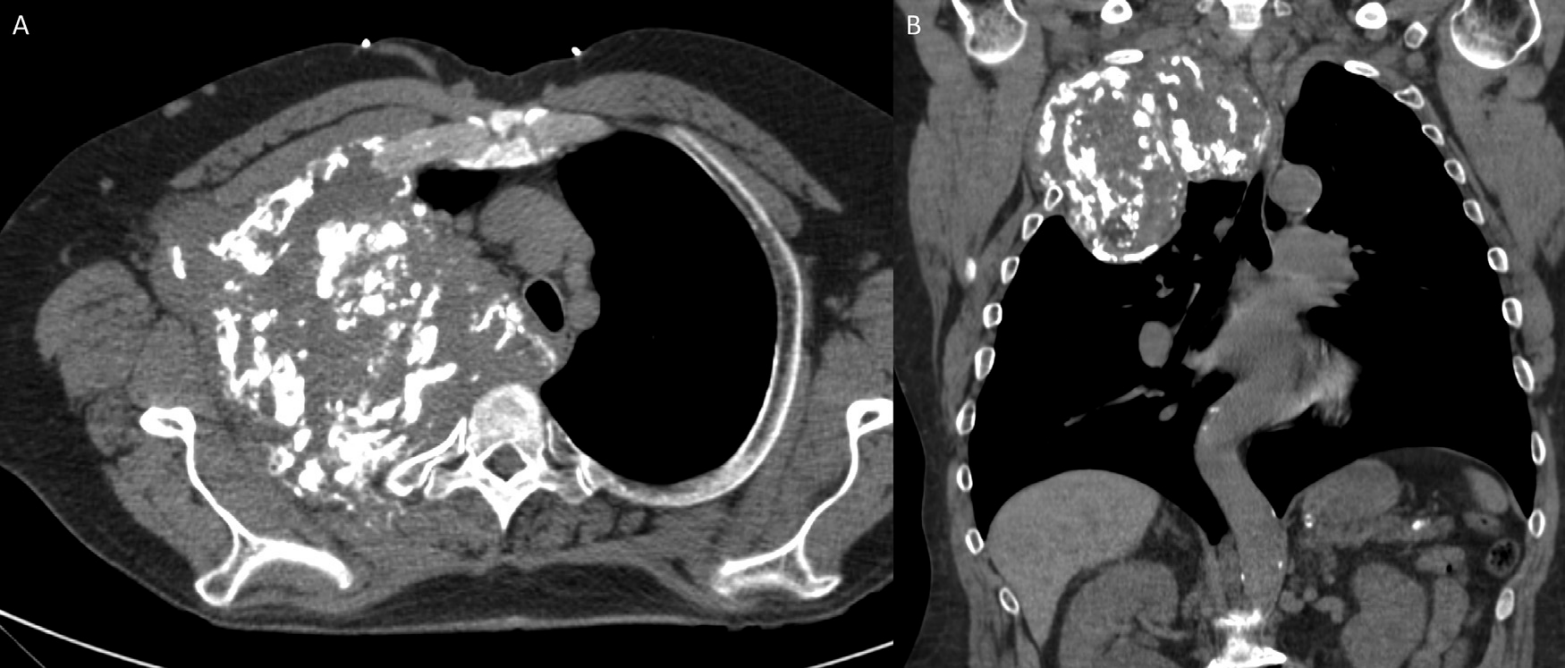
Unenhanced CT of the chest, axial (A) and coronal (B) demonstrates a solid soft tissue mass in the right upper chest wall measuring 13.3 × 15.6 × 13.4 cm, with prominent amorphous internal foci of calcifications. This mass resulted in mass effect on the trachea and superior mediastinal structures. This mass extends through the chest wall into the right axilla, and erodes the right second rib
